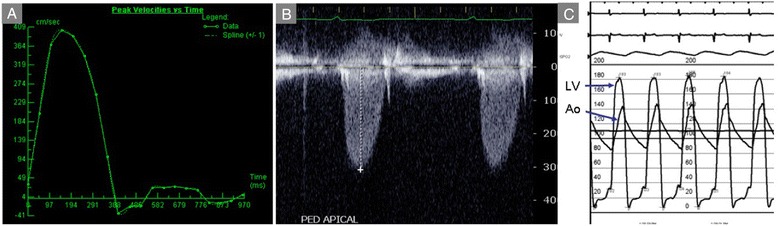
Aortic Stenosis (continued). a Quantification of phase-contrast data across the stenotic aortic valve shown in Fig. 6 indicates severe stenosis, with peak velocity of 4 m/s. b Apical continuous-wave Doppler recording in the same patient underestimates stenosis severity (3 m/s) due to misalignment relative to the direction of stenotic flow. c Invasive hemodynamics confirmed severe stenosis, with simultaneous aortic (Ao) and left ventricular (LV) pressure measurement translating to an aortic valve area of 0.44 cm^2^/m^2^, with <0.5 cm^2^/m^2^ considered critical AS
